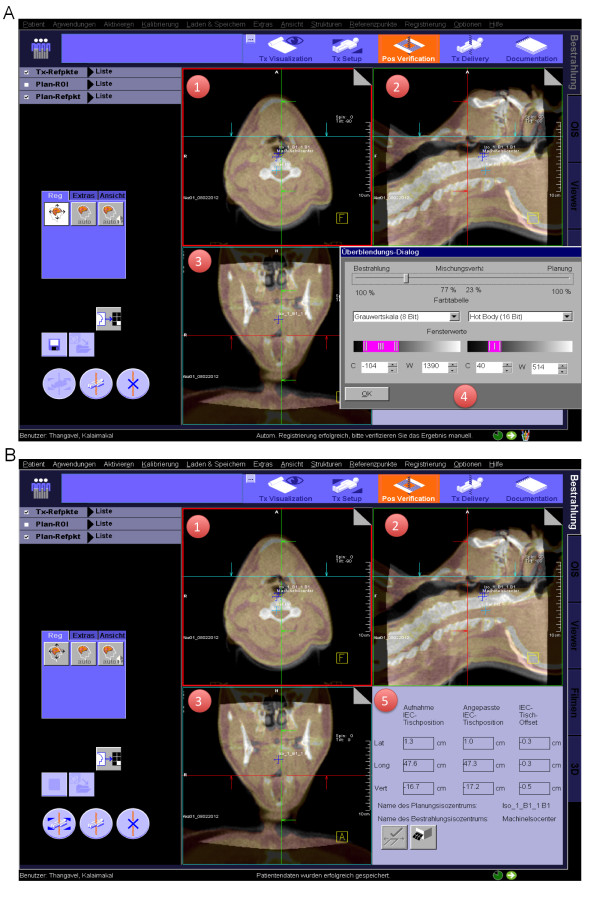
a/b: Routine photon therapy position correction tool: 1: axial cone-beam CT/planning CT scan overlay; cone-beam CT: gray scale, planning CT: hot body 2: sagittal cone-beam CT/planning CT scan overlay 3: coronal cone-beam CT/planning CT scan overlay 4: slider to switch between the x-ray (position control) and DRR (planning scan). 5: resulting correction vector.
